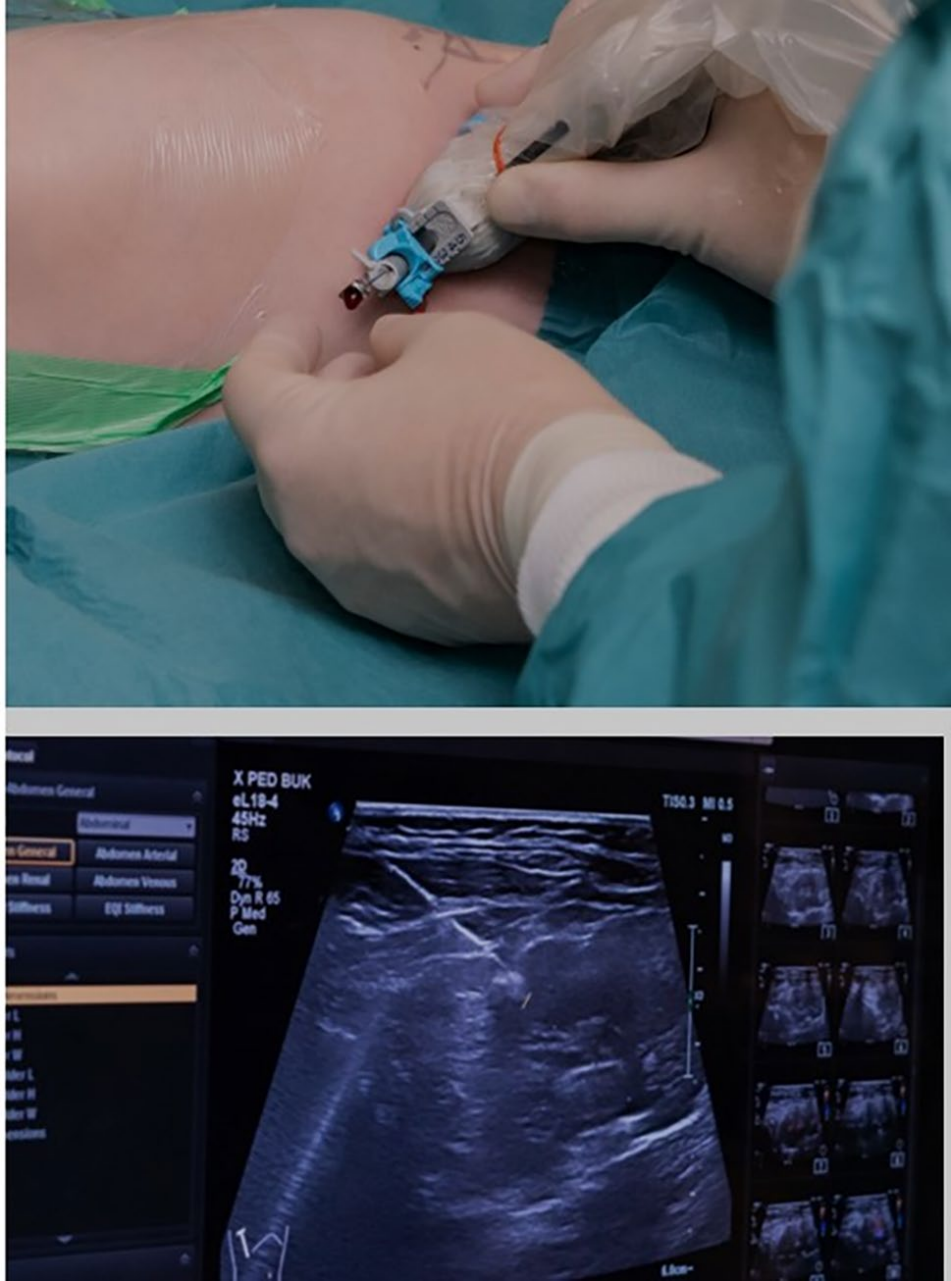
Ultrasound-guided percutaneous insertion of the distal catheter
